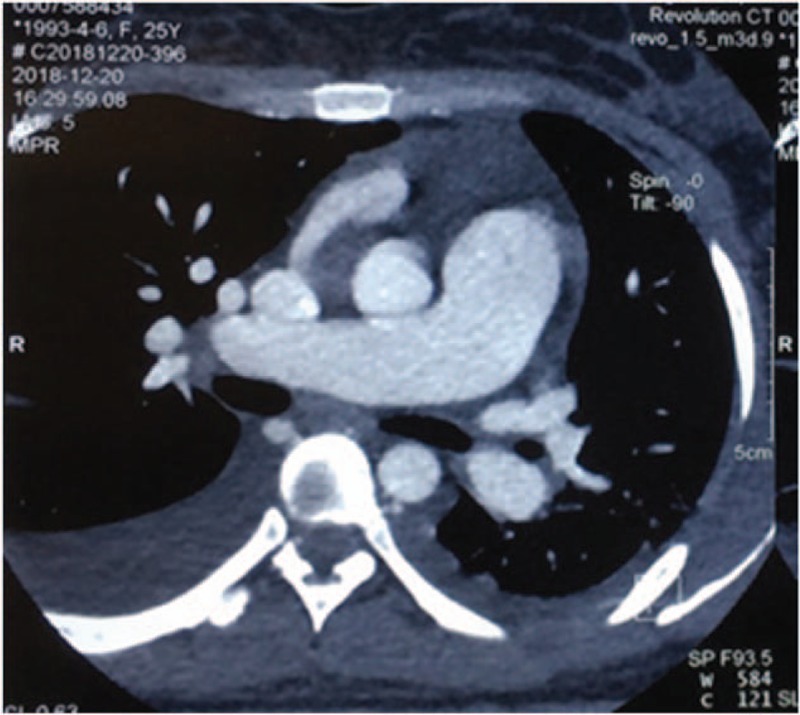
Computed tomography chest with contrast, axial cut shows markedly dilated pulmonary trunk 29 mm.
